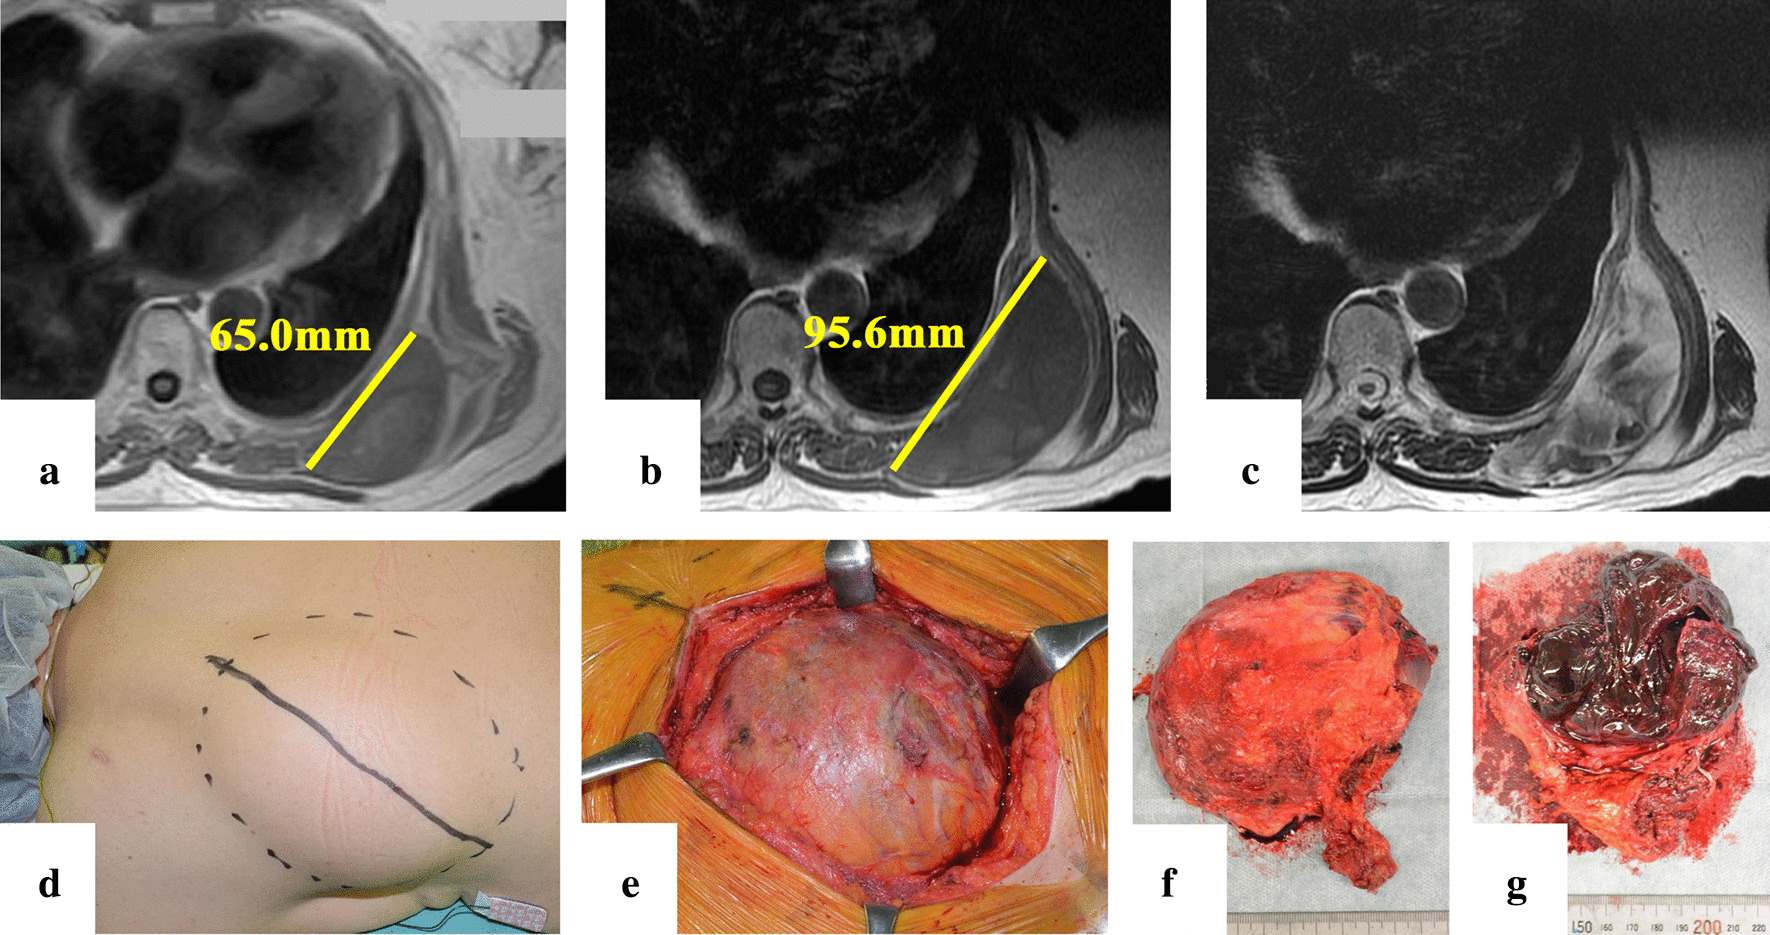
a T1-weighted magnetic resonance imaging (MRI) image 2 months before the surgery. The tumor size was 65 mm. b T1-weighted MRI image 2 days before the surgery. The inside of the tumor exhibited low-signal intensity, while an increase in size was observed (95.6 mm) compared with that 2 months before. c T2-weighted image of the same layer shown in b. The inside of the tumor exhibited heterogeneous high-signal intensity. d Protuberant tumor on the patient’s back. e and f Resected tumor and a membrane with a smooth surface covering the tumor. g Section of the resected tumor. The tumor was filled with blood clots
